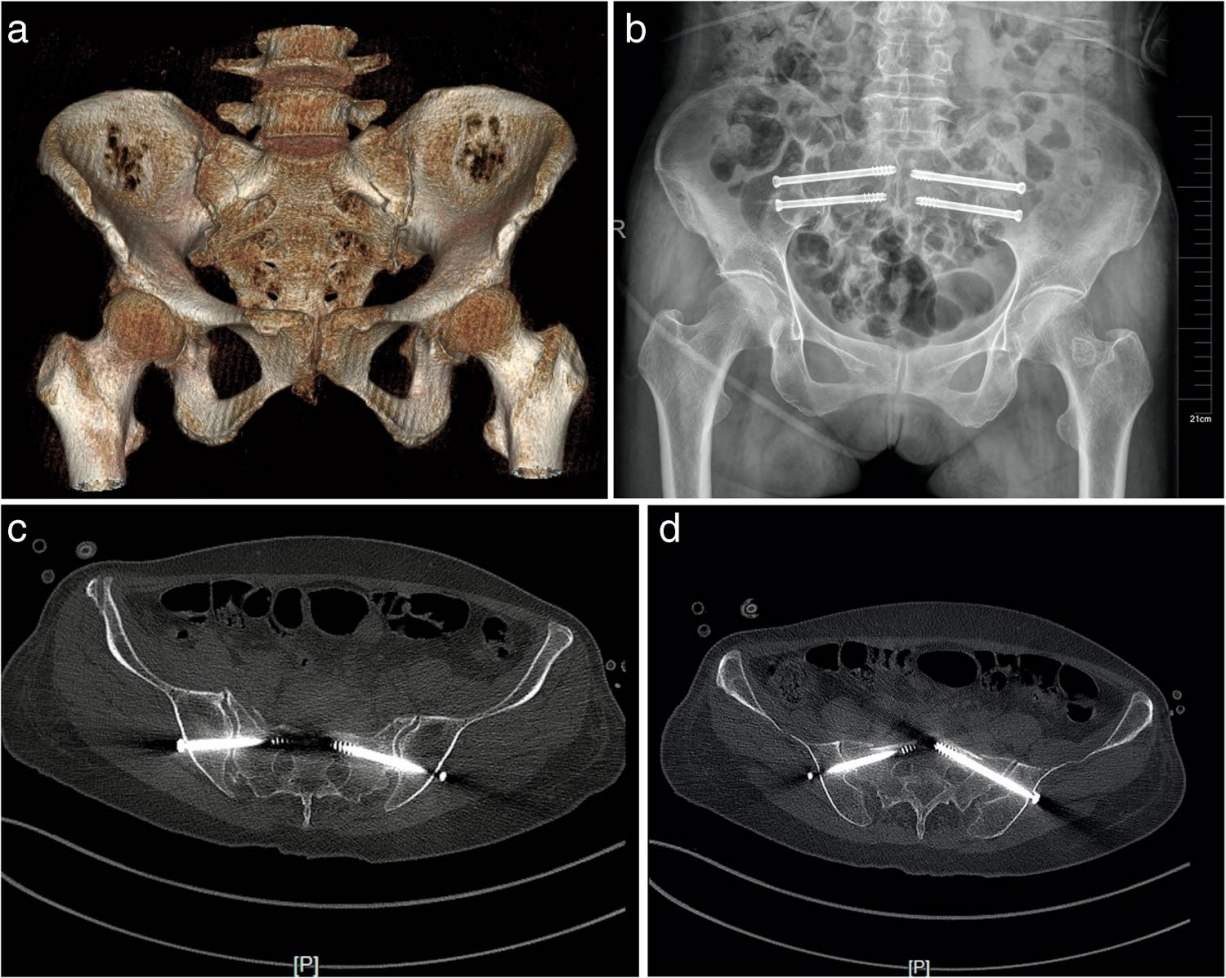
a 67-year-old female patient with bilateral sacroiliac joint injury; a CT scan pre-operation; b X-ray in post-operation; c post-operation CT scan showed that the S1 segment screws were well fixed; d post-operation CT scan indicates accurate implantation of S2 segment screw
